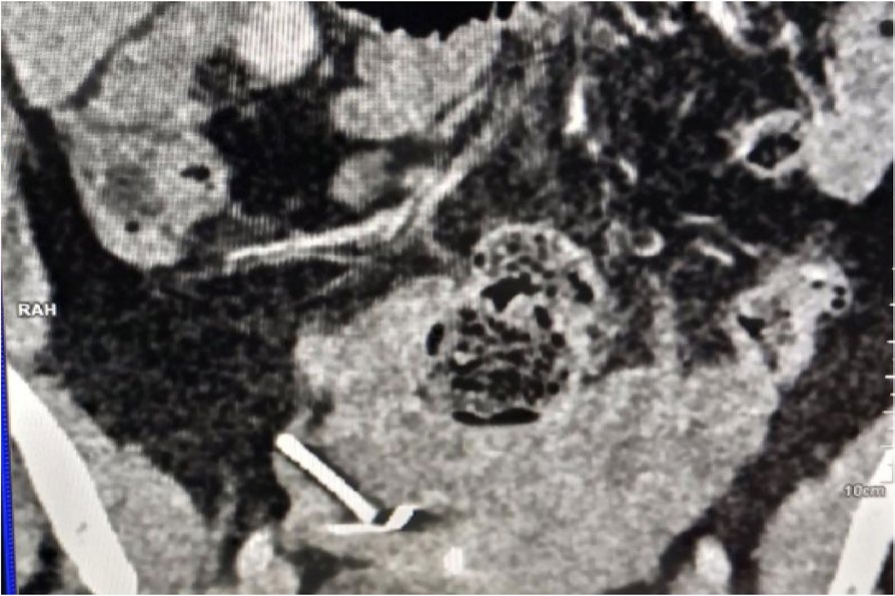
CT Scan of the Abdomen with Inverted T
